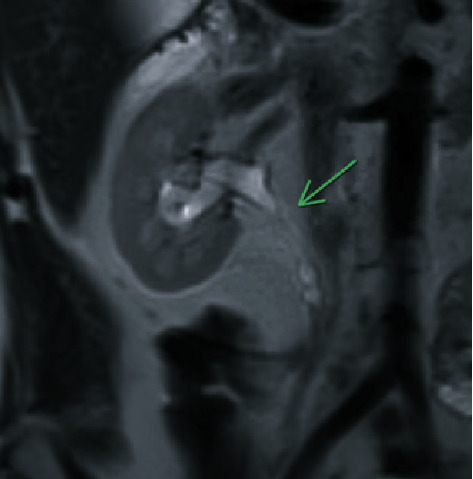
MRI shows encasement of the proximal right ureter, which intraoperatively was found to be due to a fibrous sheath.
